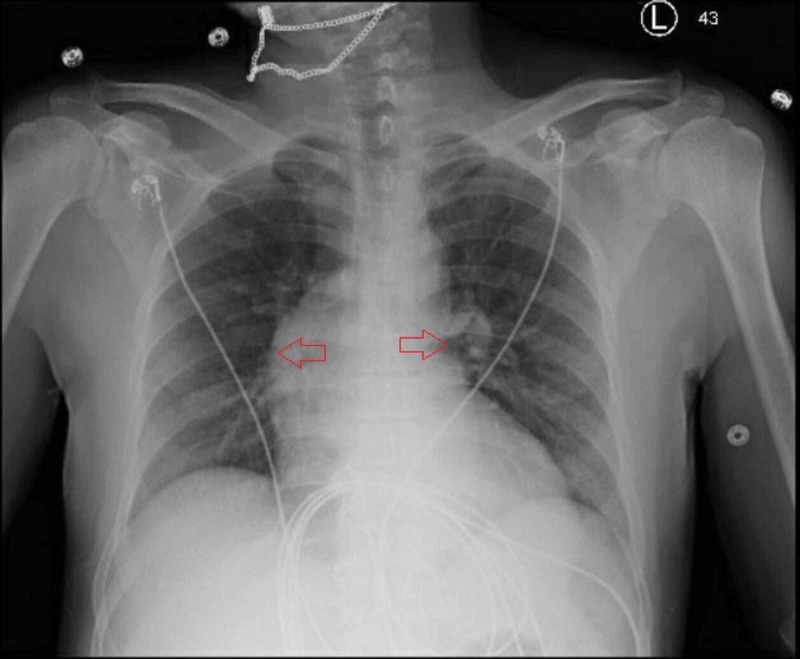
Chest X-ray showing clear lungs and a prominent mediastinum (red arrows).
